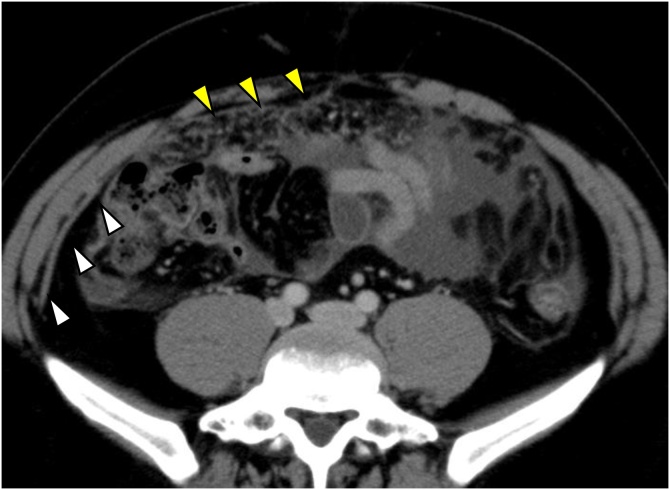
Chest-abdominal computed tomography showing ascites fluid, and panniculitis with peritoneal nodules (white arrows) and the thicken of the omentumn(yellow allows).
